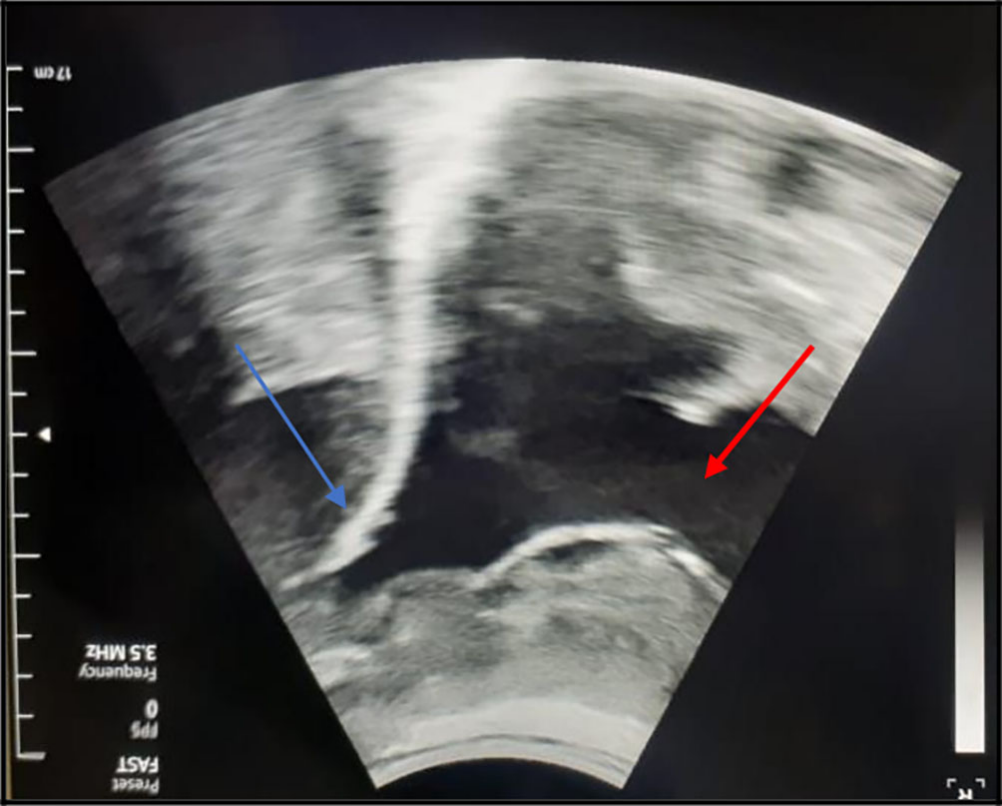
The chest ultrasound showed a massive, anechoic, free pleural effusion (red arrows) in the left side associated with a multinodular pleura (blue arrows).
